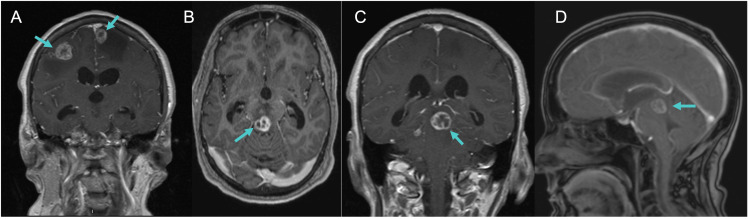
Contrasted MRI brain demonstrating bilateral frontal lesions and a lesion in the dorsal midbrain. (A) Coronal view of T1 post-gadolinium sequence. Blue arrows point to two lesions in the frontal lobe. (B) Axial view T1 post-gadolinium sequence at the level of the midbrain. Blue arrow points to contrast enhancing lesion near the tectum. (C) Coronal view T1 post-gadolinium. Blue arrow points to a contrast-enhancing lesion in the midbrain. (D) Sagital view T1 post-gadolinium. Blue arrow points to an enhancing lesion in the midbrain.
